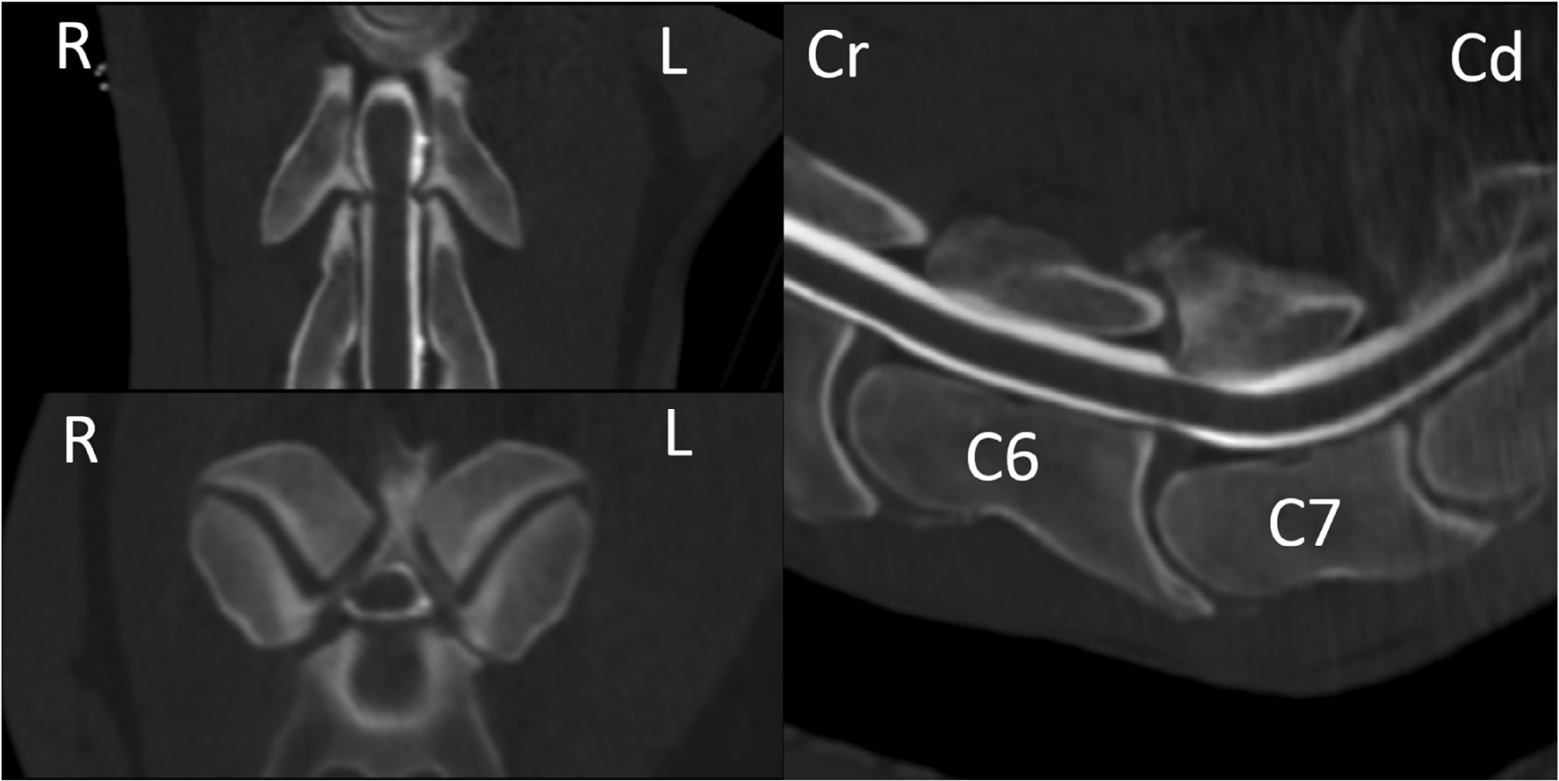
Computed tomographic (CT) myelographic 3D multiplanar reconstruction at the level of C6‐C7 in case 33. Note the profound narrowing of the contrast media column surrounding the spinal cord at this level
